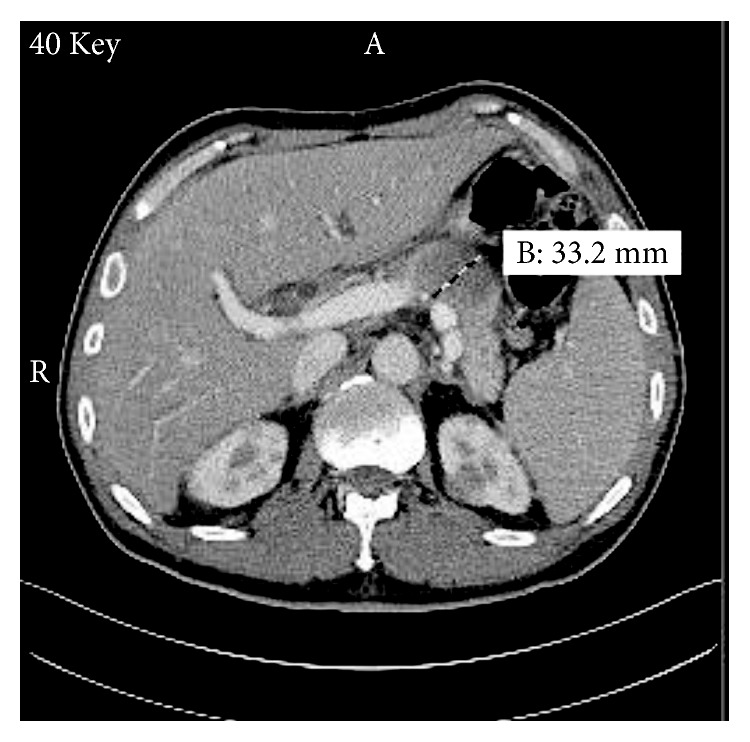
Computed tomography scan of the abdomen showing 33.2 mm mass in the pancreatic body. Multiple bilateral renal masses can also be appreciated.
